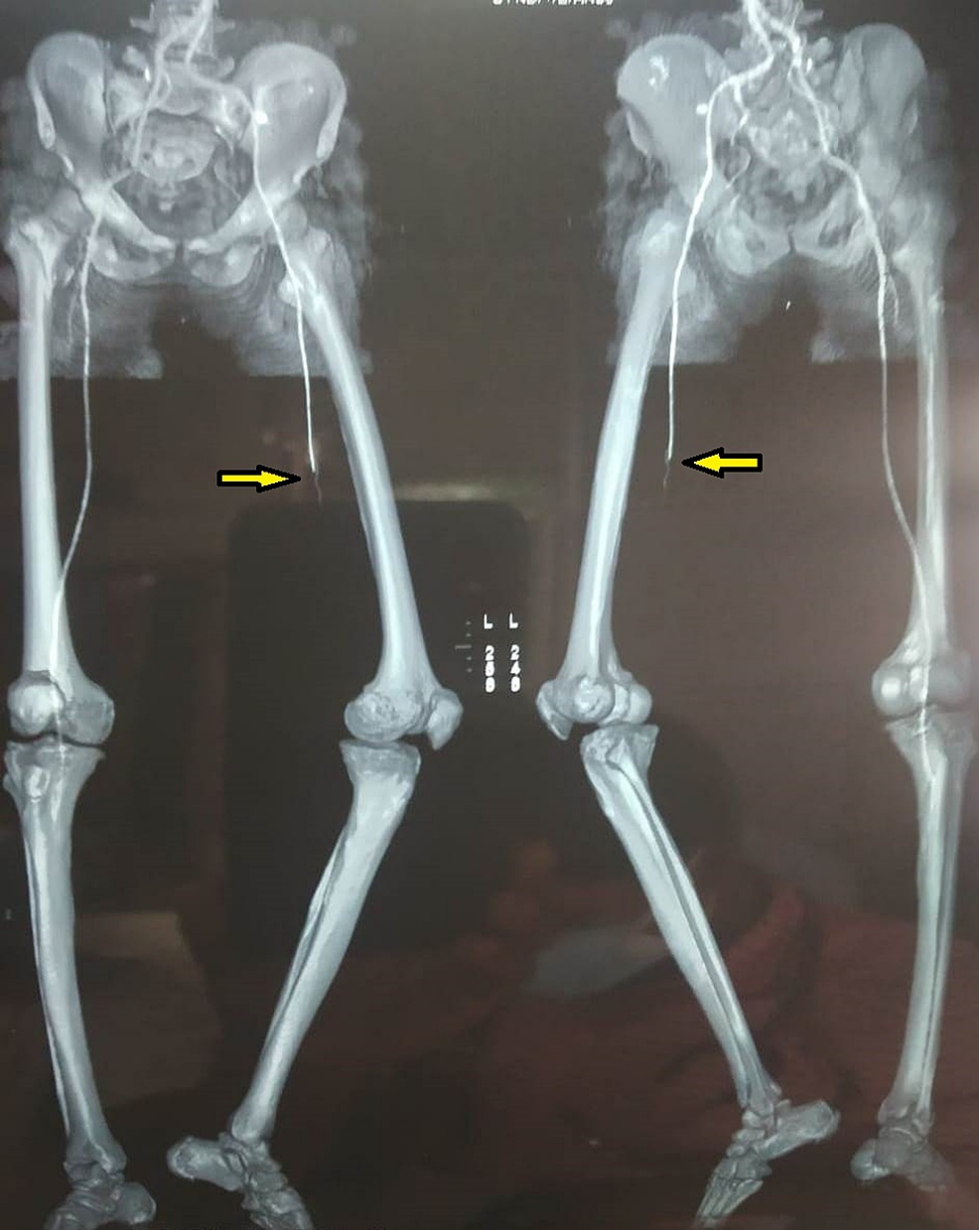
3D Virtual reconstructed CTA image showing complete blockage of the mid superficial femoral artery, popliteal artery, and infra popliteal artery with poor collateral status (Arrow). There is no distal reformation of the vessels CTA= CT Angiography
